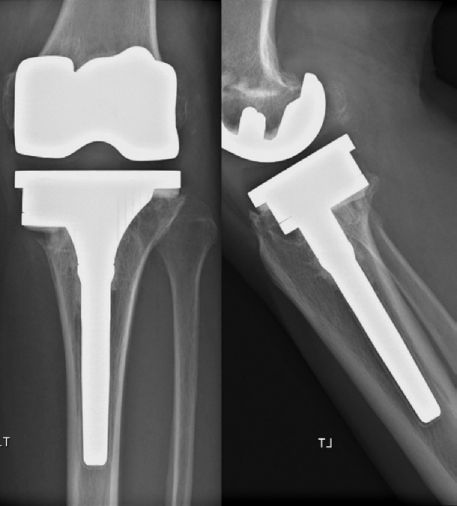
Postoperative radiographs at 5-year follow-up.
